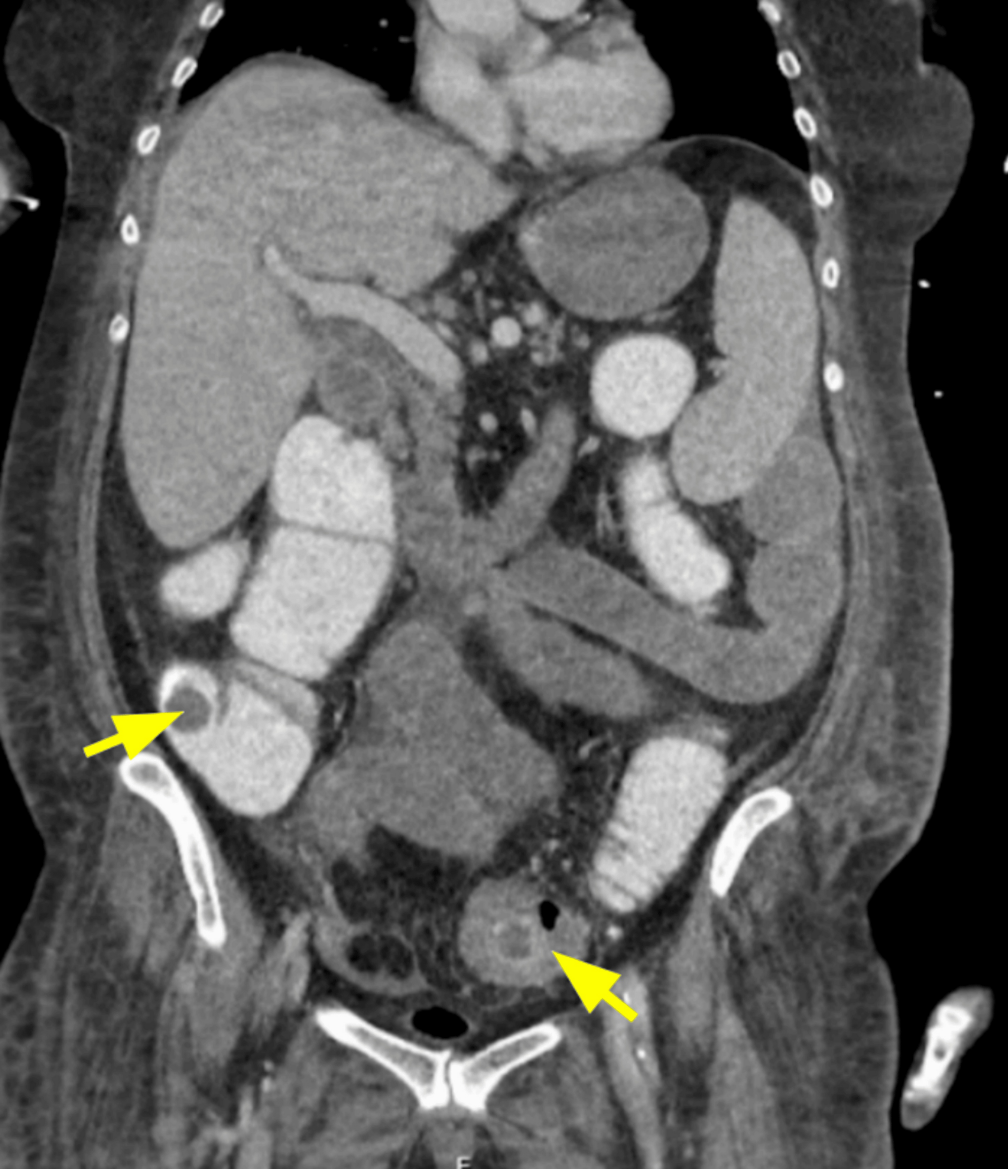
Coronal CT image of the abdomen and pelvis showing synchronous gallstones in the cecum and sigmoid colon, indicated by yellow arrows
